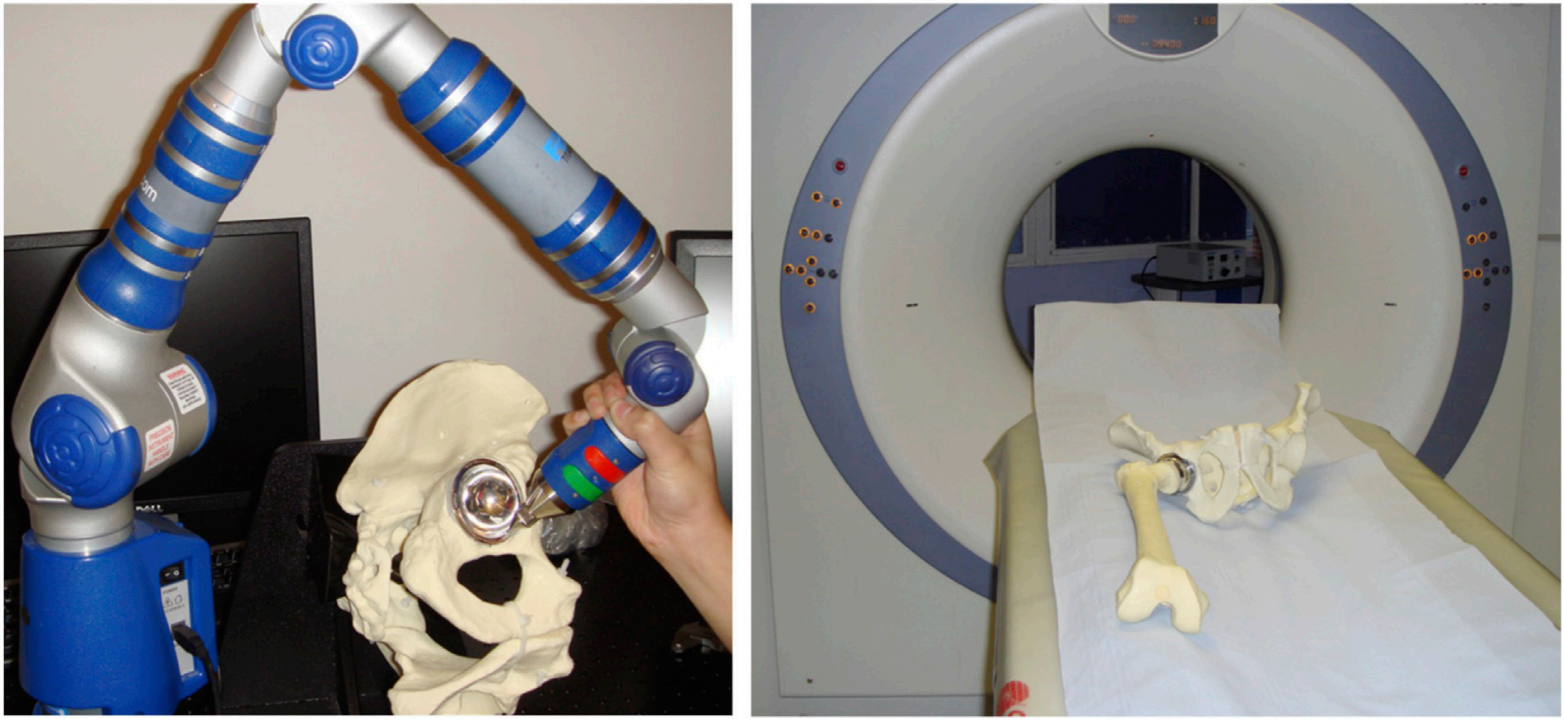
(Left) The orientation of the metal acetabular cup implanted in the pelvic Sawbone model being measured by the digitising arm. (Right) The model placed on the CT scanner table with the fiducials (hexagonal fully threaded titanium screws) placed on landmarks for robust landmarking.
